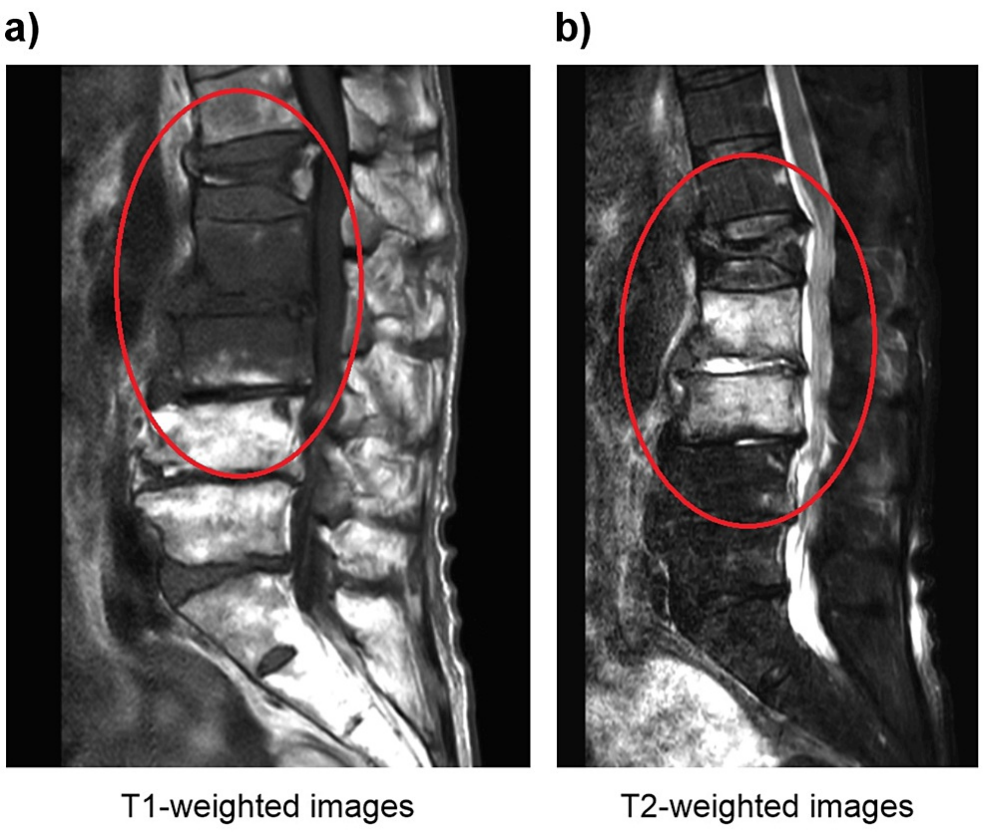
Magnetic resonance imaging without contrast of the lumbar spine Magnetic resonance imaging without contrast of the lumbar spine showing fluid accumulation in the L2/3 and L3/4 discs and (a) low-signal changes in the L2 and L3 vertebrae on T1-weighted imaging and (b) high-signal changes in the L2 and L3 vertebrae on T2-weighted imaging. An old compression fracture can be seen in L1 (red circles)
